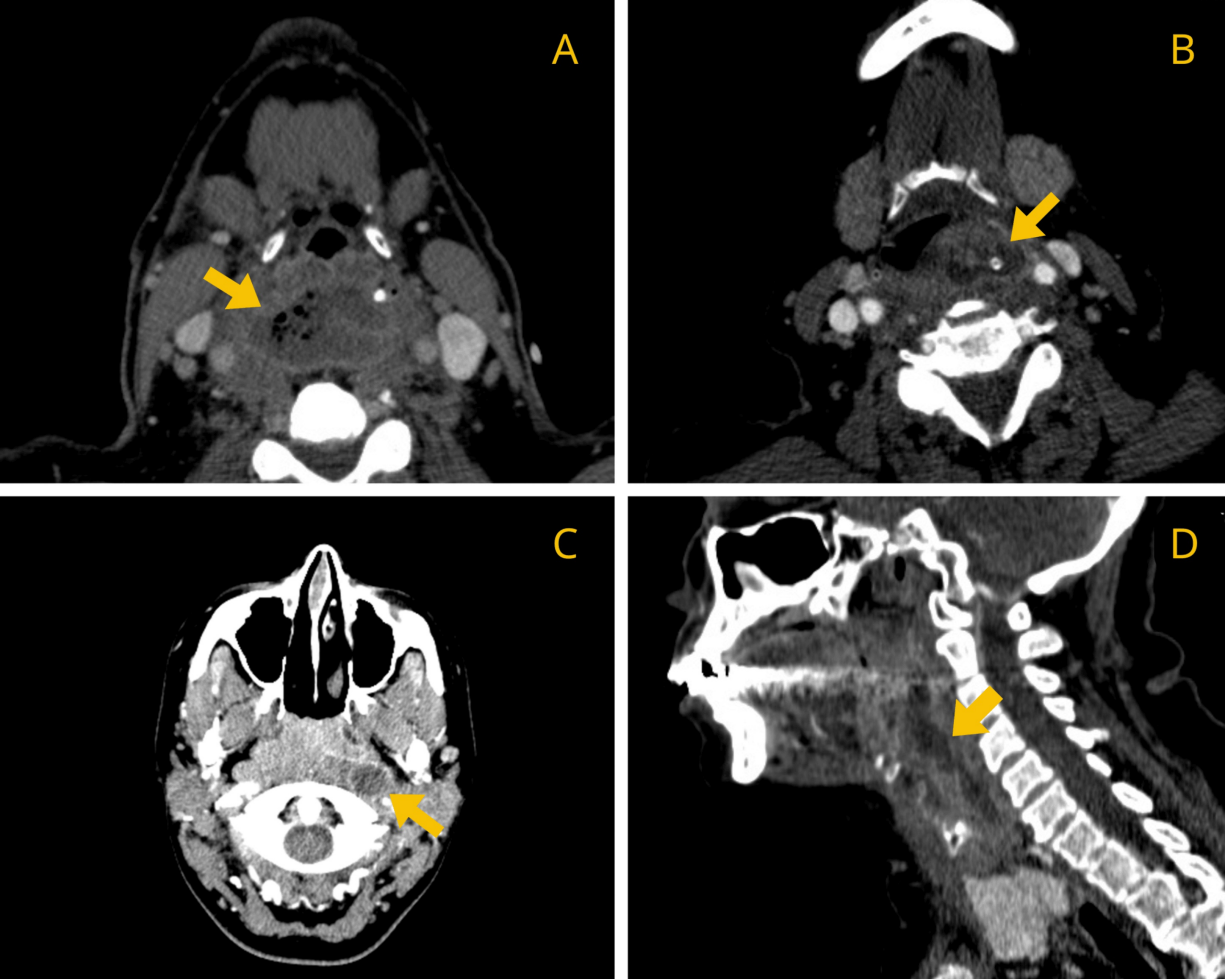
Contrast-enhanced CT images of patients 1 (A), 3 (B), 6 (C), and 9 (D) demonstrating RPA (yellow arrow). RPA: retropharyngeal abscess.
